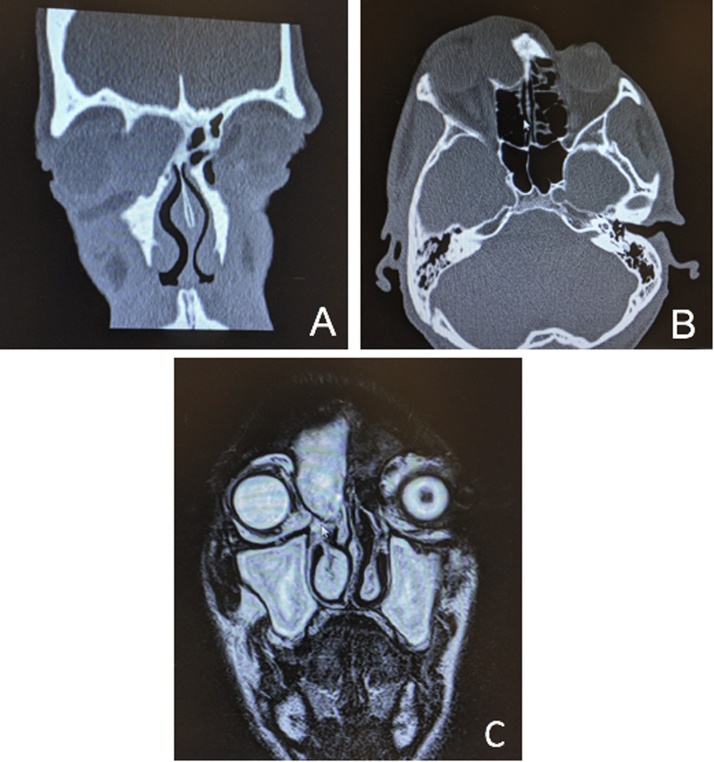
(A) Axial and (B) coronal plane of computed tomography scan showing a frontal sinus mucocele with intra-orbital extension. (C) Coronal view of contrast-enhanced magnetic resonance highlighting a benign fluid-filled lesion in the frontal sinus.
